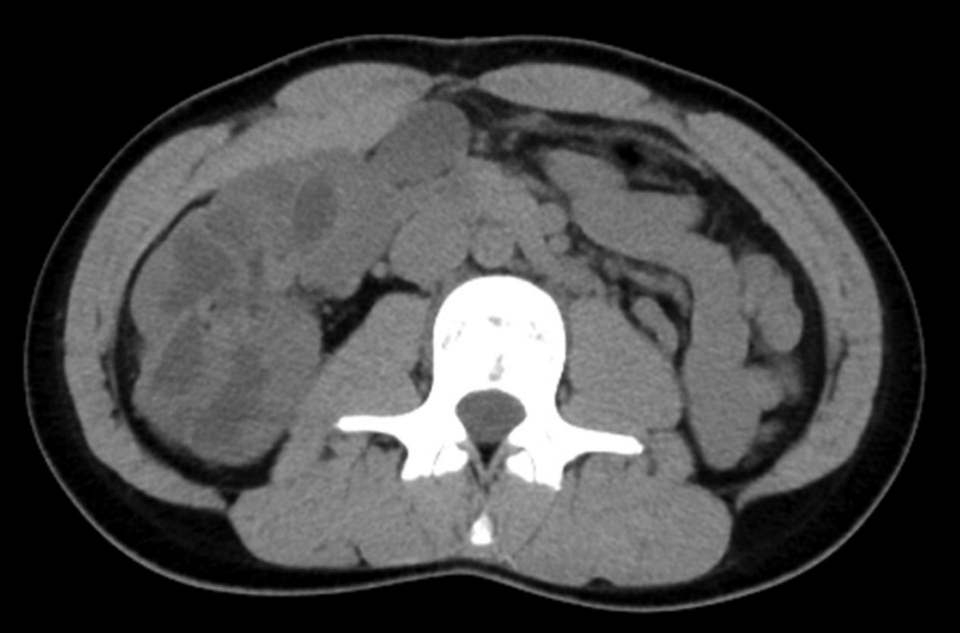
Abdominal CT performed 12 months after the left pyeloplasty. The axial view reveals shrinking of the right renal pelvis and calyx.
